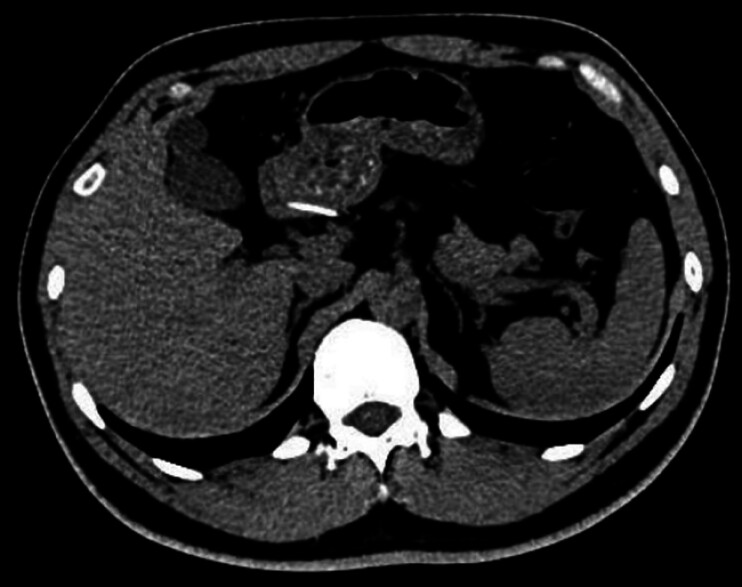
Abdominal computed tomogram showing a linear hyperdense foreign body adjacent to the lesser curvature of the gastric antrum.
